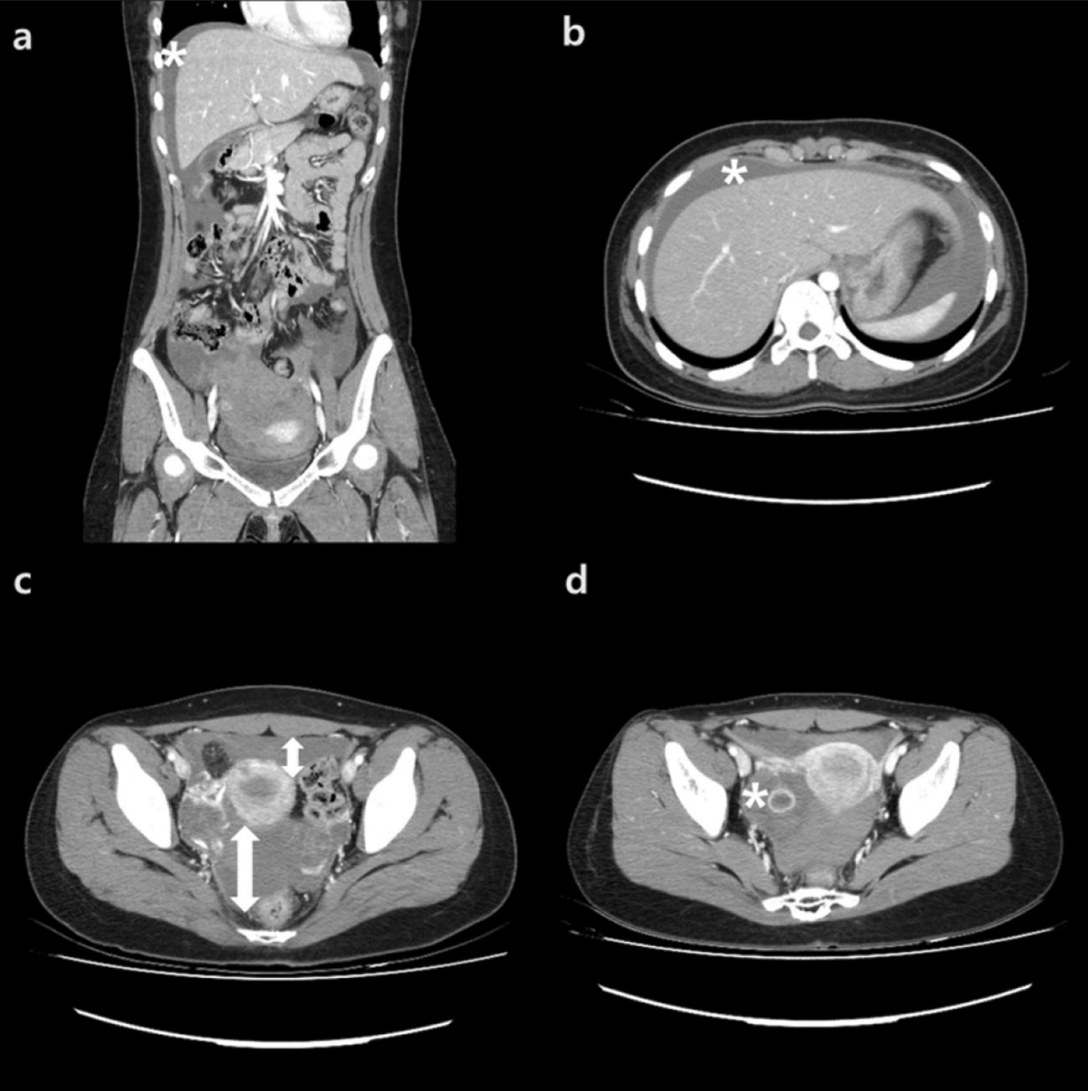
Abdomen–pelvic CT. (a) In coronal view, there is a liver-dome fluid collection (*). (b) In transverse view, fluid collection around liver-dome and stomach (*). (c) In transverse view, methods of anterior cul-de-sac fluid collection depth measurement, posterior cul-de-sac fluid collection depth measurement. (d) Extravasation from right ovary cyst rupture, hyperechogenic ring formation (*).
